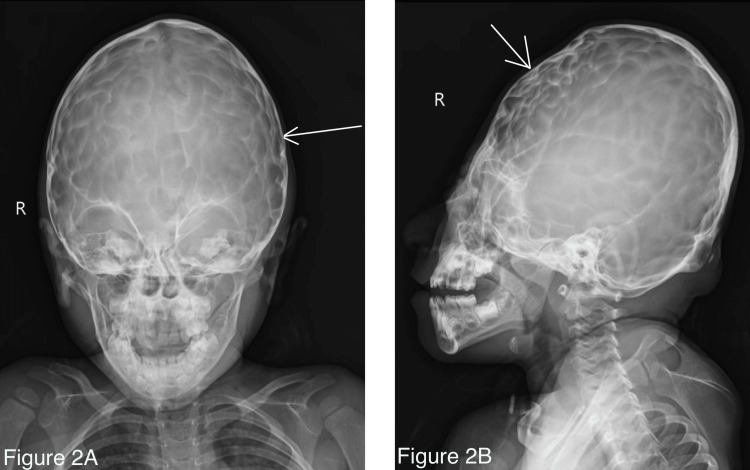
Radiographs of the patient's skull A: Anteroposterior view showing convolutional markings of the gyri suggestive of copper beaten skull appearance. B: Lateral view showing indentations on the skull suggestive of copper beaten skull appearance involving the whole skull secondary to long-standing increased intracranial pressure due to craniosynostosis due to Crouzon syndrome.
